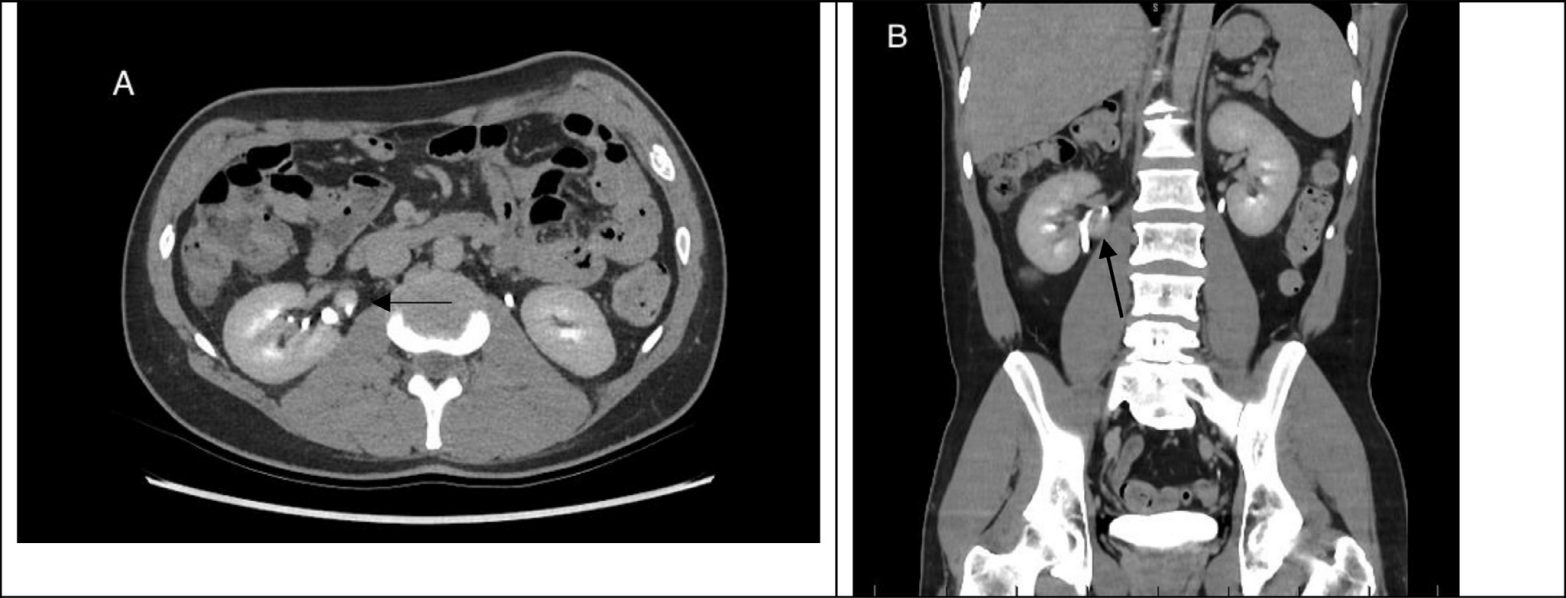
Axial image of computed tomography of the abdomen (A) demonstrating the collecting system (arrow) of the right supernumerary kidney with the upper calyx draining into the main ureter with the right Kidney (B).
